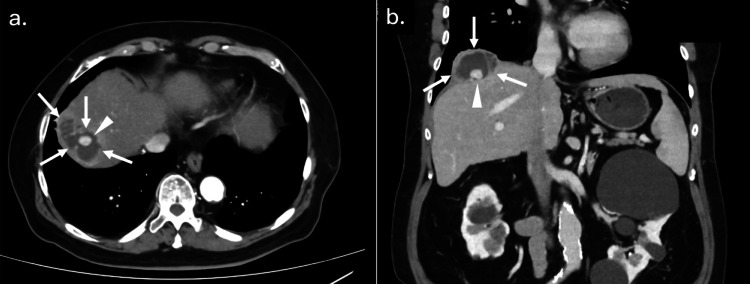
Abdominal CT scan findings six months after the initial surgery (a, b) Abdominal CT, six months after laparoscopic cholecystectomy, revealed an encapsulated fluid retention (arrows) compatible with an abscess containing a calcified lesion (arrowheads) in the right subphrenic space.
